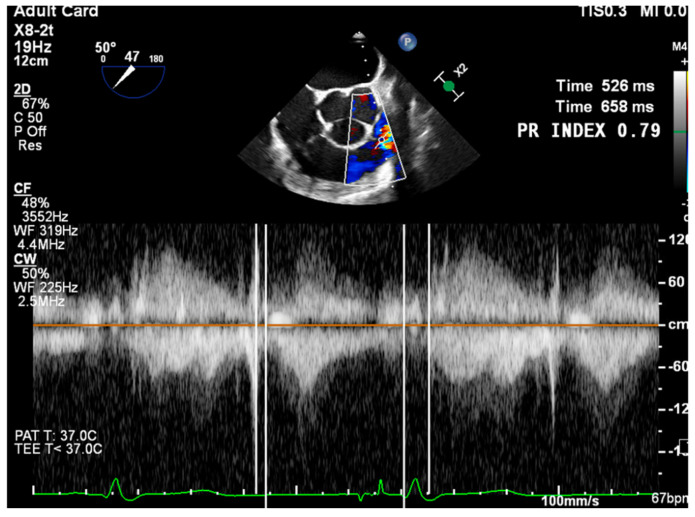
ME RV Inflow/Outflow with continuous wave Doppler (CWD) and angle correct, capturing the spectral Doppler profile of the PR jet. PR duration is measured from its onset in early diastole to the end of the regurgitant Doppler signal. The total diastolic time is measured from the end of the forward pulmonary flow (coinciding with the onset of the retrograde PR flow) to the beginning of the next forward pulmonary flow curve.
